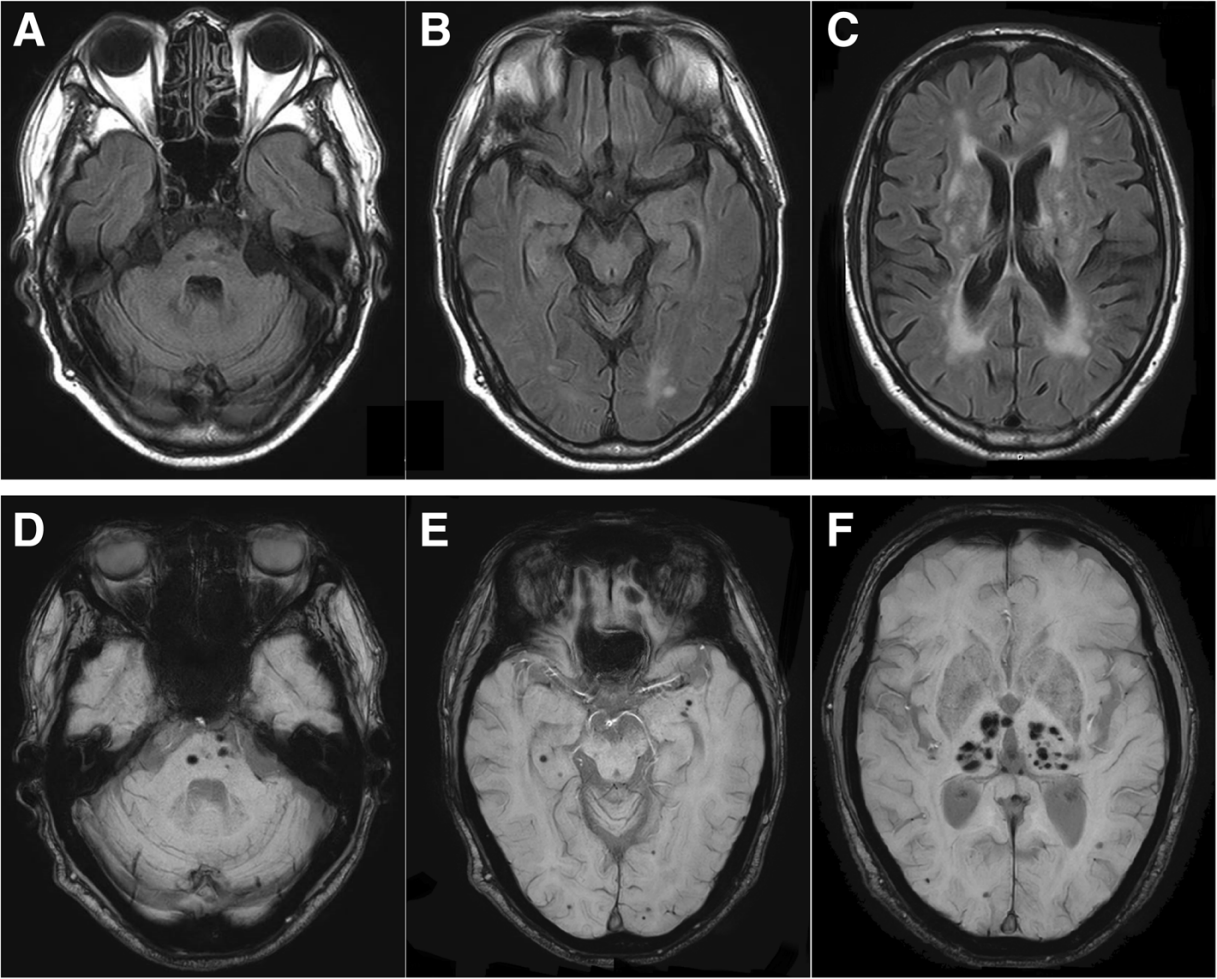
Brain-MRI findings of our patient. a–c Axial FLAIR image showing the bilateral ischemic lesions in pons, periventricular white matter and deep white matter. d–f: Axial susceptibility-weighted imaging (SWI) showing multiple micro-bleeding lesions in pons, bilateral subcortical white matter and bilateral thalamus
